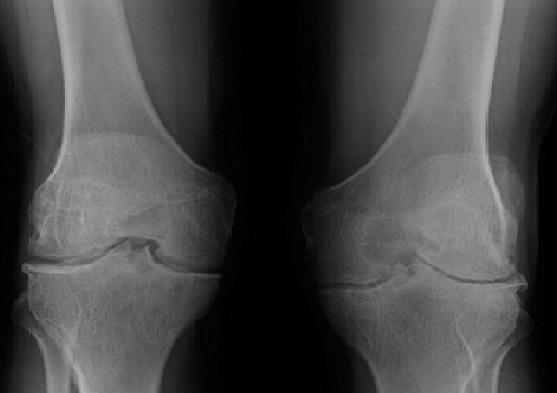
Homme 32 ans, hémophile B mineur. Radiographie comparative des genoux en incidence de face montrant des géodes des plateaux tibiaux et des condyles fémoraux associées à un épointement des épines tibiales et pincement de l'interligne fémoro-tibiale bilatérale: arthropathies évoluées
